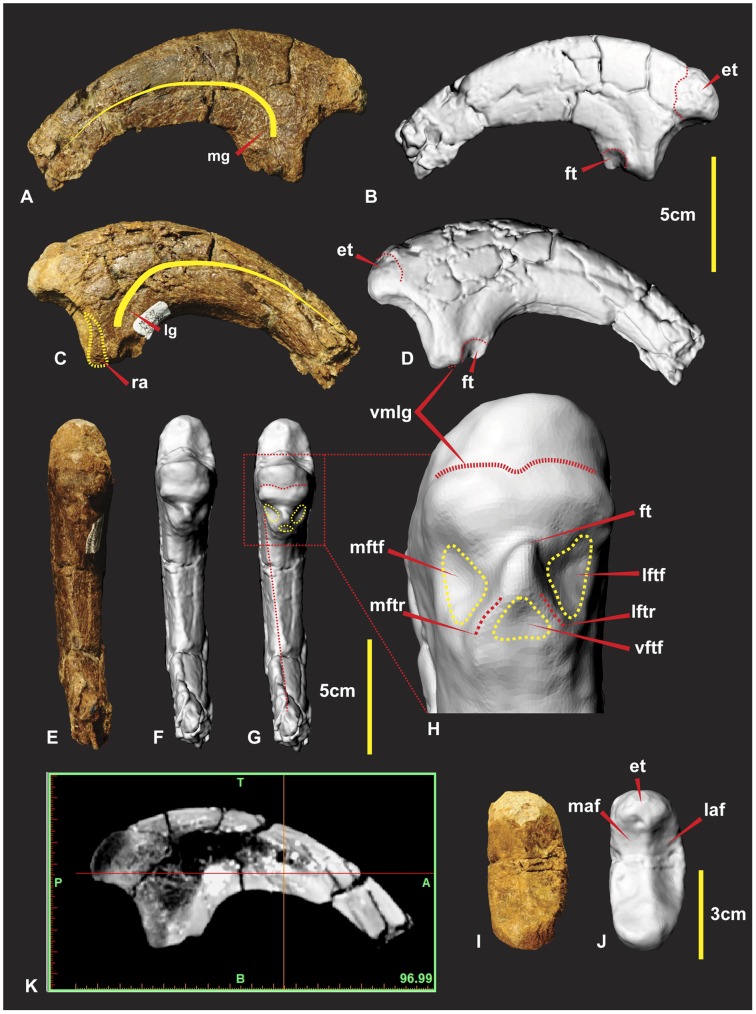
Right manual phalanx I-2. Right manual phalanx I-2 in lateral (A & B), medial (C & D), flexor tendon close up (E), ventral (F & G), proximal (H & I), central proximo-distal cross-section CT image (J). *Abbreviations:* et, extensor tubercle; ft, flexor tubercle; laf, lateral articular facet; lftf, lateral flexor tubercle facet; lftr, lateral flexor tubicle ridge; lg, lateral groove; maf, medial articular facet; mftf, medial flexor tubercle facet; mftr, medial flexor tubicle ridge; mg, medial groove; ra, rugose area; vmlg, ventral medio-lateral groove;;; vftf, ventral flexor tubercle facet.
